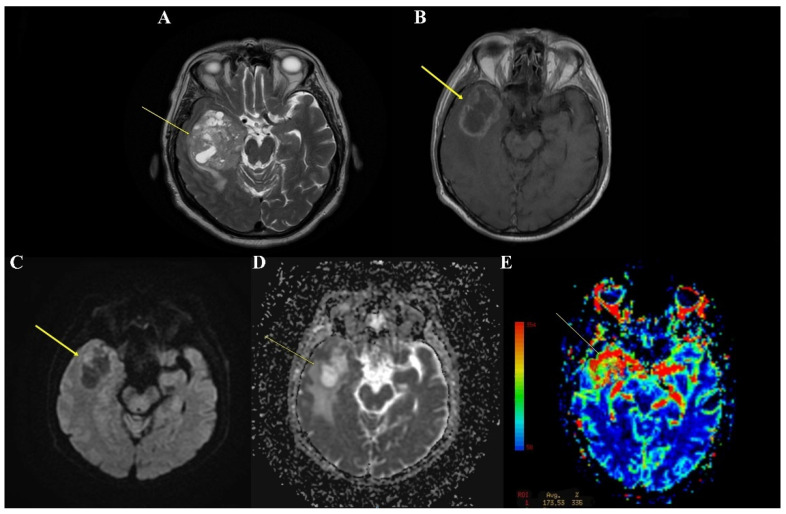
(A) MRI axial T2 weighted image reveals a right temporal lobe mass, with a solid/cystic aspect, with maximum axial dimensions of 65/59 mm, surrounded by edema. (B) MRI axial T1-weighted with contrast highlights a right temporal lesion with peripheral contrast enhancement. (C,D) MRI axial DWI and ADC sequences show diffuse restriction in the anterior aspect of the lesion. (E) MRI axial PWI shows an increase in rCBV at the lesion level.
